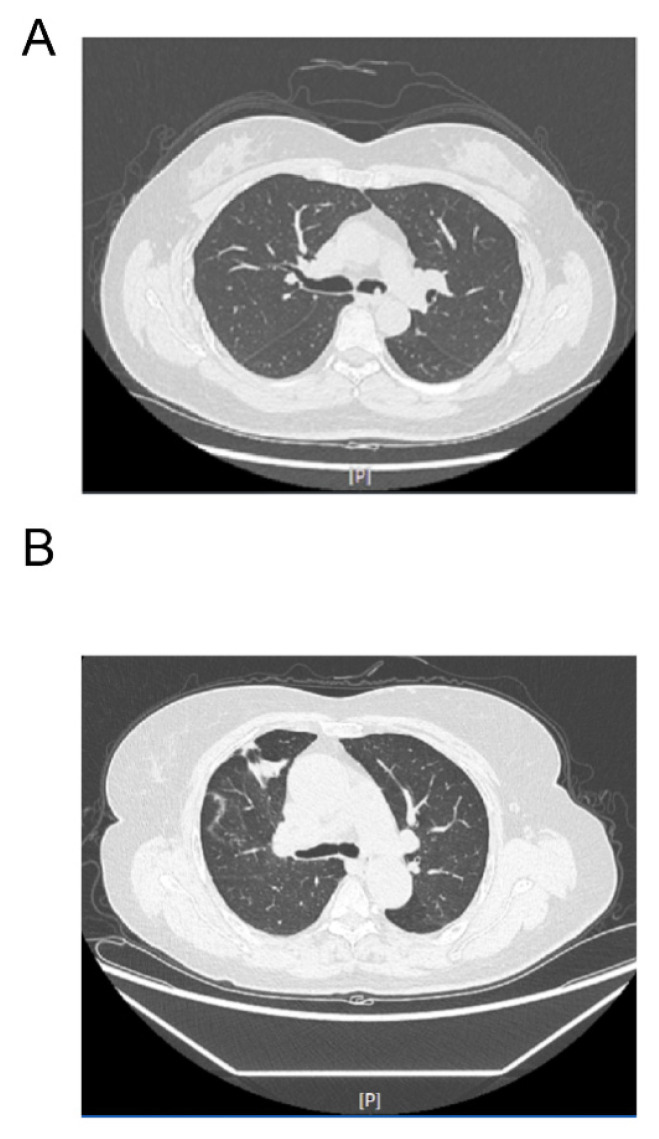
Representative thoracic CT images showing endobronchial lesions and secondary pulmonary changes: (A) Axial contrast-enhanced CT image demonstrating an intraluminal polypoid lesion within the airway, with associated peripheral secondary inflammatory consolidation in the right lung. (B) Axial CT image demonstrating an endobronchial mass causing localized obstruction in the right bronchial tree. (window settings: [P], pulmonary window).
